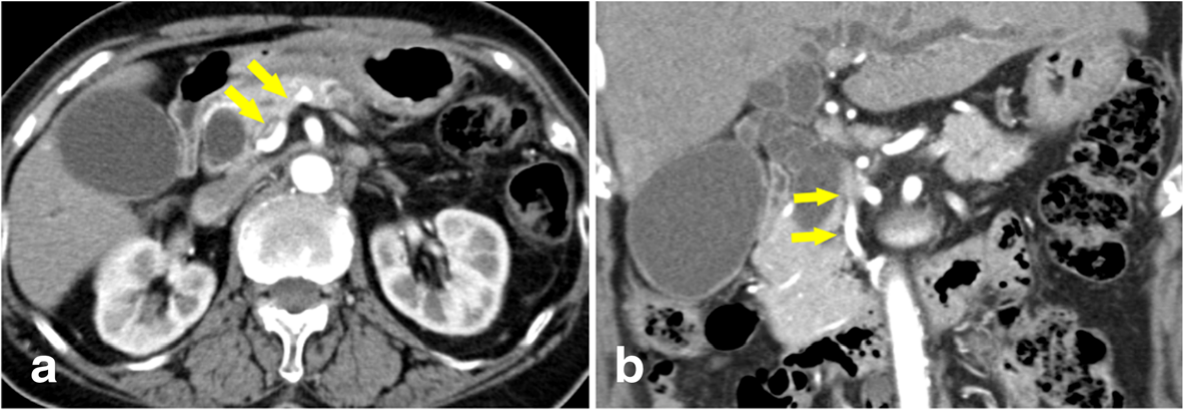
a, b Computed tomography image showing the arc of Buhler
